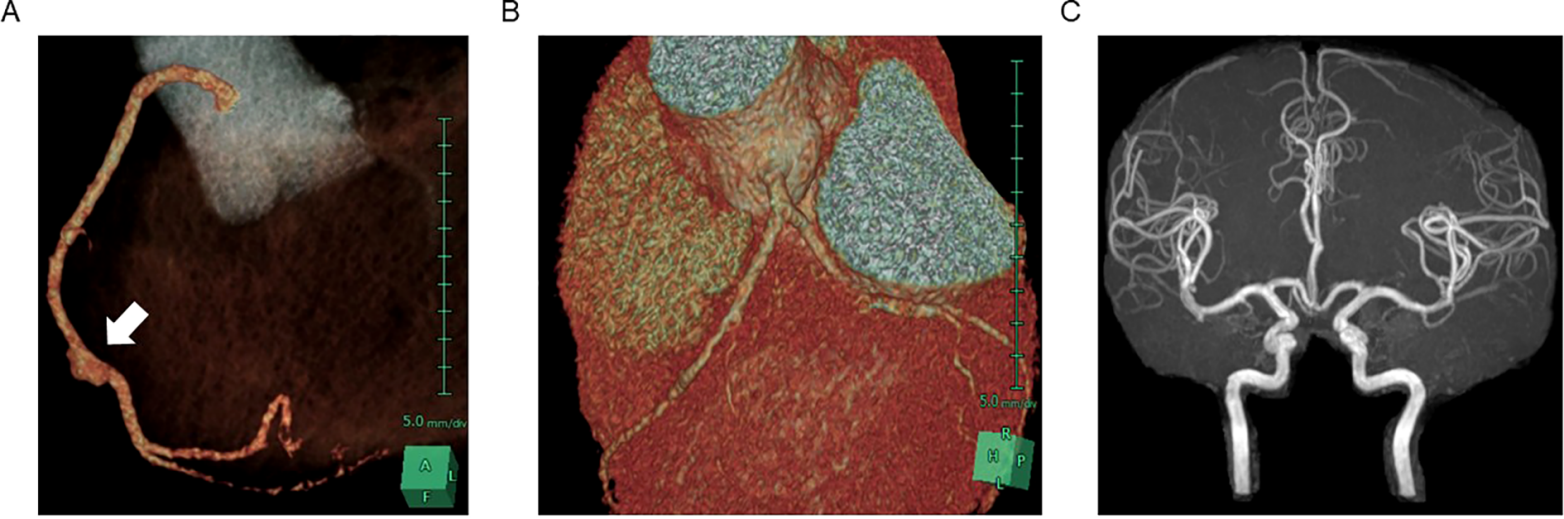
CT image of asymptomatic pseudoaneurysm in the right coronary artery. 3D reconstruction of contrast-enhanced CT image in coronary arteries demonstrated pseudoaneurysm in right coronary artery (A) and no dilation observed in left coronary arteries (B). MR angiography showed no dilation in cerebral arteries (C).
